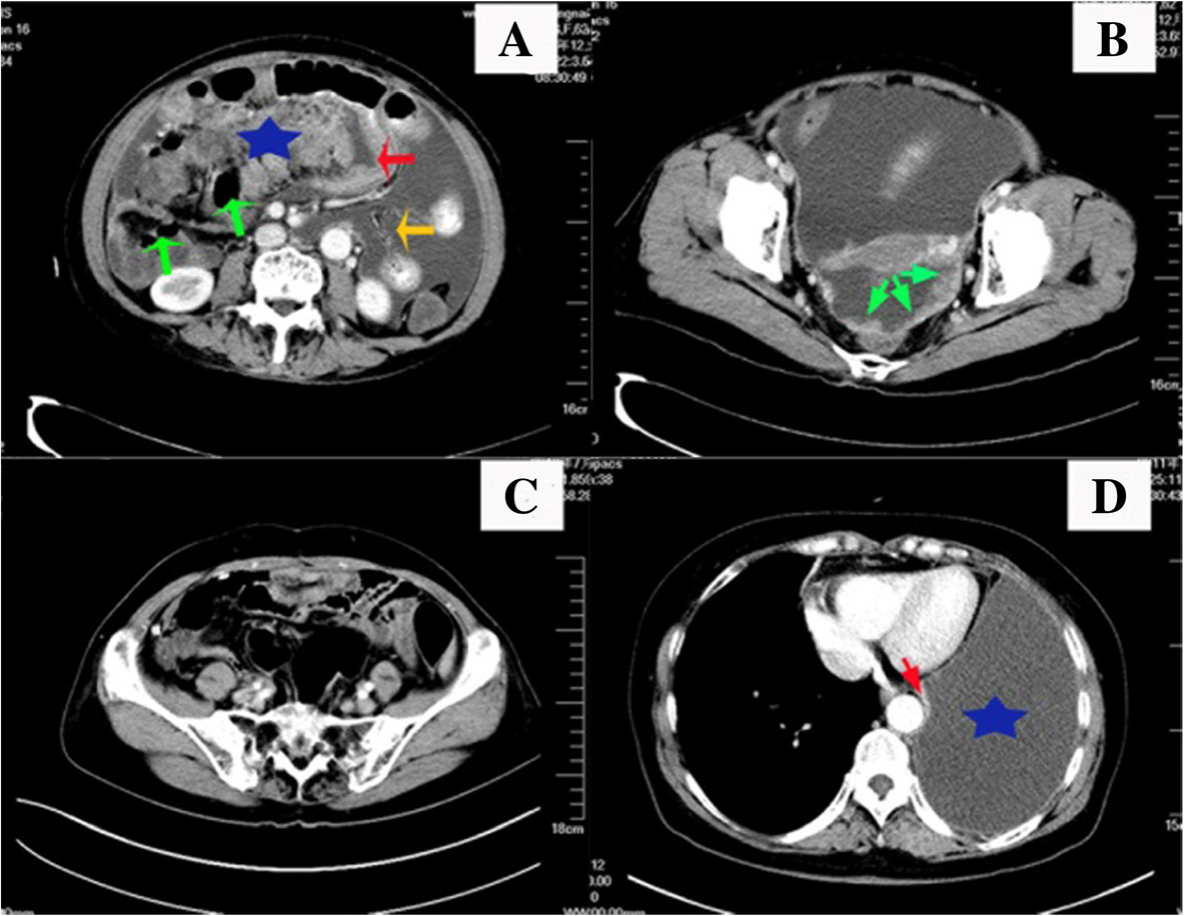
a Intravenous and oral contrast-enhanced CT scan shows ascites (*orange arrow*) and omentum cake (*star*) which partially encases intestinal tract (*green arrows*) and adheres to and presses the stomach (*red arrow*). b The pelvic peritoneum thickening (*green arrows*), no ovarian and regional lymph nodes involvement was found. c No abdominal recurrence when lung metastasis was found. d Massive left pleural cavity effusion (*star*) and atelectasis left lower lobe (*red arrow*)
